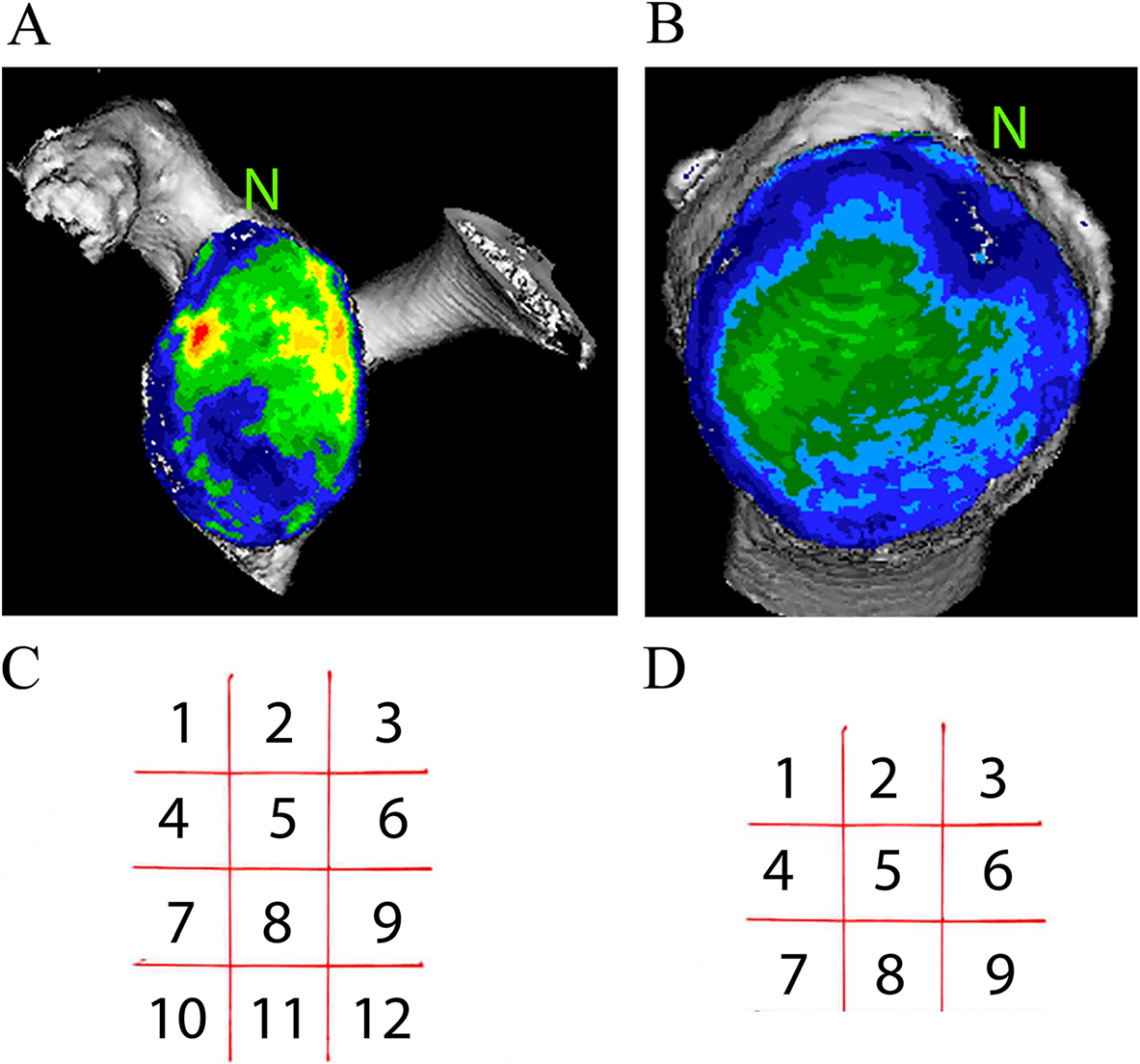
Representative CT-OAM images showing mineral distribution of (A) glenoid, and (B) humeral head. CT-OAM false colour-encoded (black > red > orange > yellow > bright green > dark green > blue > violet, see: [57]) and superimposed on the identical 3-D-reconstructed joint. N points (A) to the most superior point of the glenoid, and (B) to notch in the humeral head formed by the tendon of the long head of biceps. (C, D) A grid was superimposed over the CT-OAM and morphology images to separate the articular surface in quadrants of glenoid (C) and humeral head (D) similar to previous work [57, 58]
